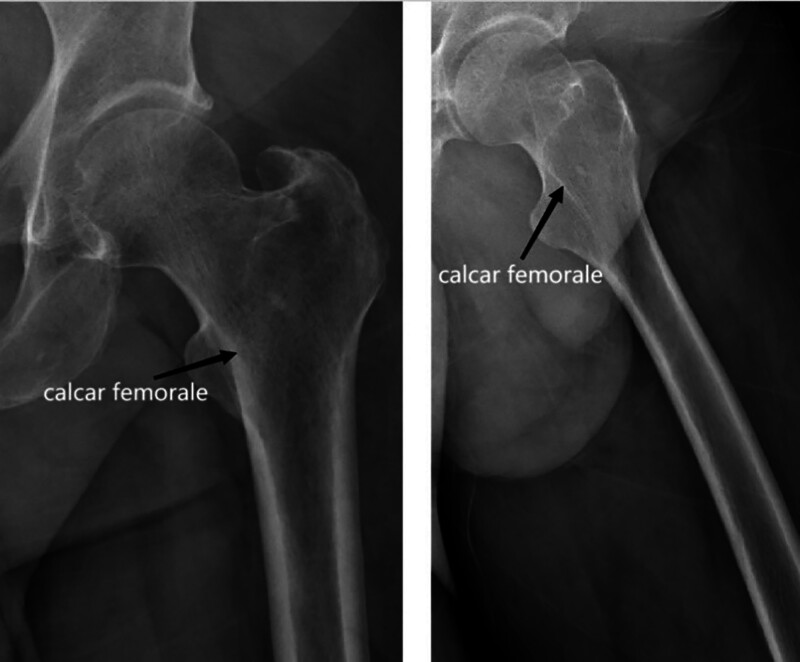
The calcar femorale in X-rays of the proximal femur at different angles.
